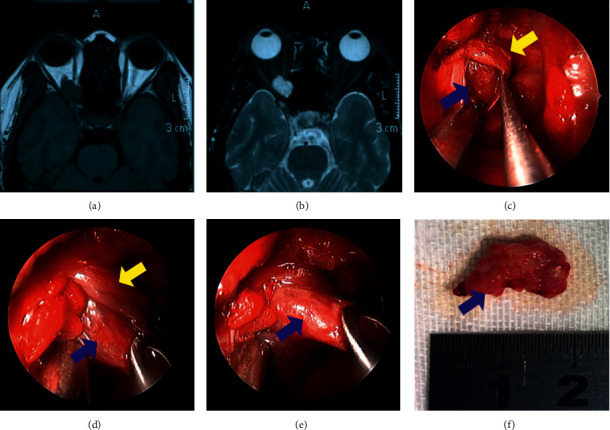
Magnetic resonance imaging of a patient with cavernous hemangioma of the orbital apex and surgical removal process. (a) T1-weighted images showed an isointensity mass in the right orbital apex. (b) Axial T2-weighted images showed a high-intensity mass. (c–f)The surgical removal of the cavernous hemangioma: the yellow arrow indicates the muscle and the blue arrow indicates the tumor.
